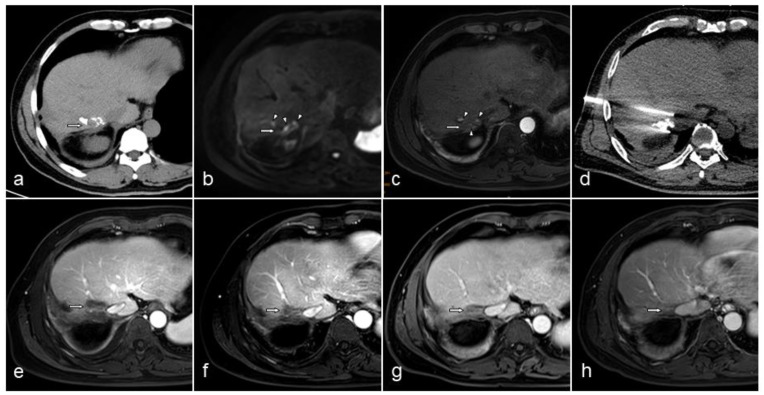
A 55-year-old male patient with hepatocellular carcinoma showing vessel patency after irreversible electroporation (IRE). Eight years ago, the chronic hepatitis B-infected liver mass was detected, surgically resected, and pathologically diagnosed to be hepatocellular carcinoma. Radiofrequency ablation and transarterial chemoembolization (TACE) was performed 4 years and 1 month ago, respectively, for tumor recurrence. IRE was then performed as the lesion was found active. (a) Computed tomography (CT) showed iodide deposition in the lesion 1 month after TACE. (b) Diffusion-weighted imaging showed a high signal in the lesion after TACE (arrow). (c) Contrast-enhanced T1-weighted image (T1WI) showed partial enhancement in the arterial phase (arrowhead). (d) CT image during the IRE procedure showed the probes in place and ablation zone. (e–h) Contrast-enhanced T1WI image in the venous phase showed hepatic vein and postcava remained patent, and the lesion gradually shrank to almost invisible at 1, 3, 6, and 12 months post procedure.
